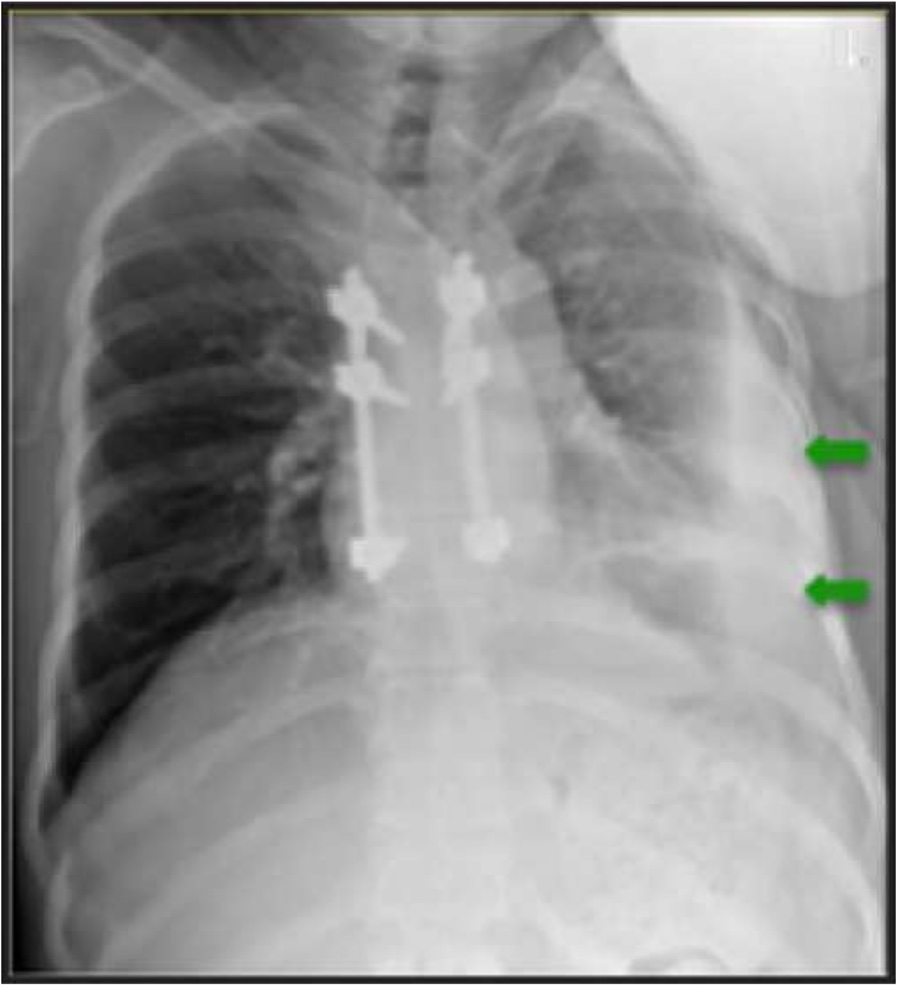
Chest radiograph in a lateral position, with pleural effusion in the left hemithorax (*arrows*)
